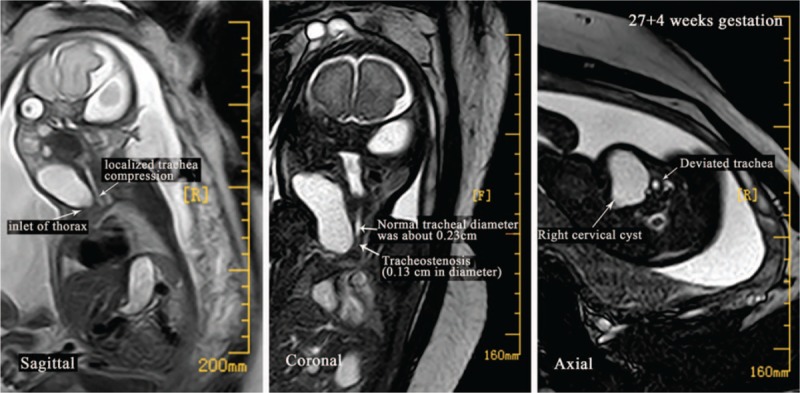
Fetal sagittal, coronal, axial MRI images at 28 weeks’ gestation (WG). MRI revealed a giant (2.2 × 4.1 × 3.6 cm) cervical cyst causing localized tracheal compression.
